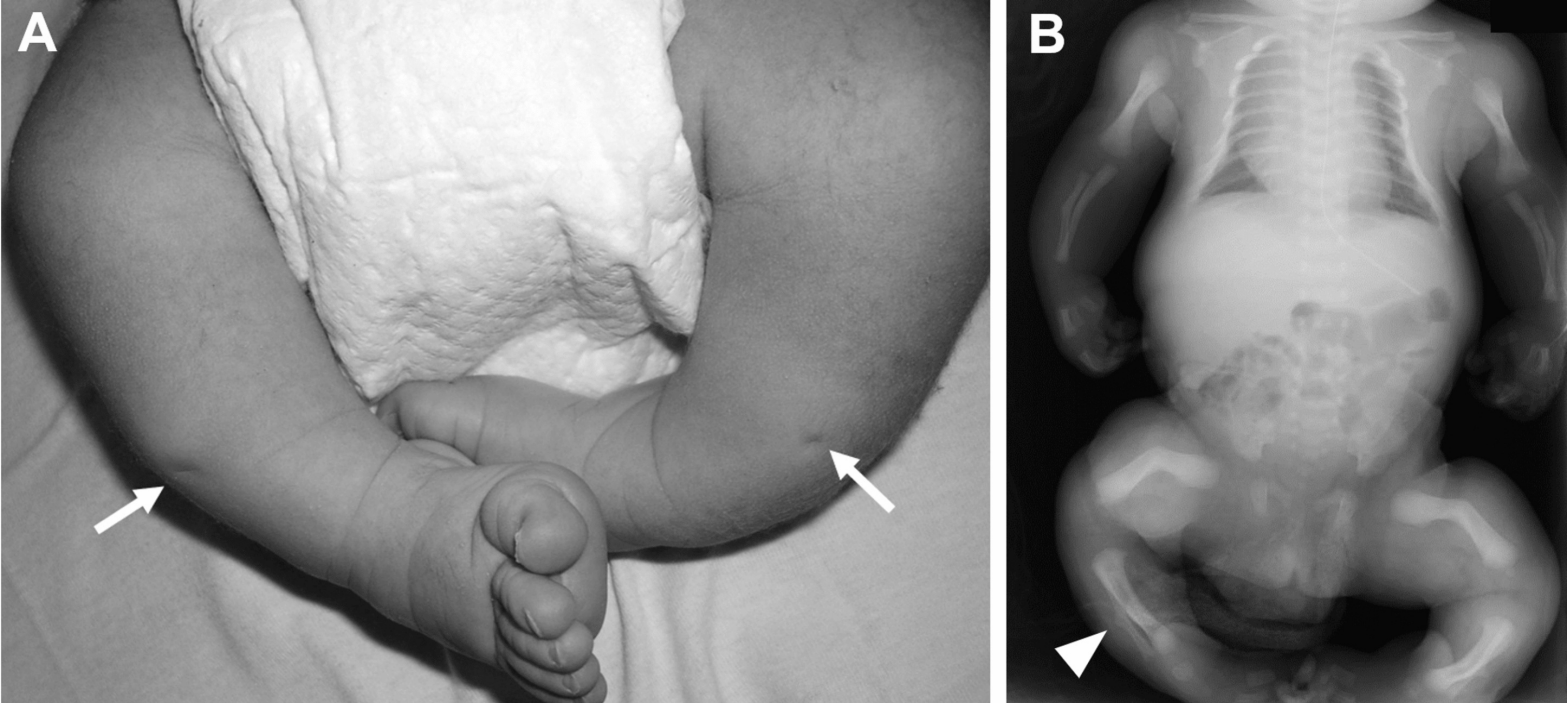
Physical and X-ray findings at birth A. Lower extremities at birth: shortening of lower limbs, the skin dimpling sign (arrow), and bilateral clubfoot. B. X-ray findings at birth: bowed radius, ulna, femur, and tibia. The bone spur of the fibula (arrowhead).
